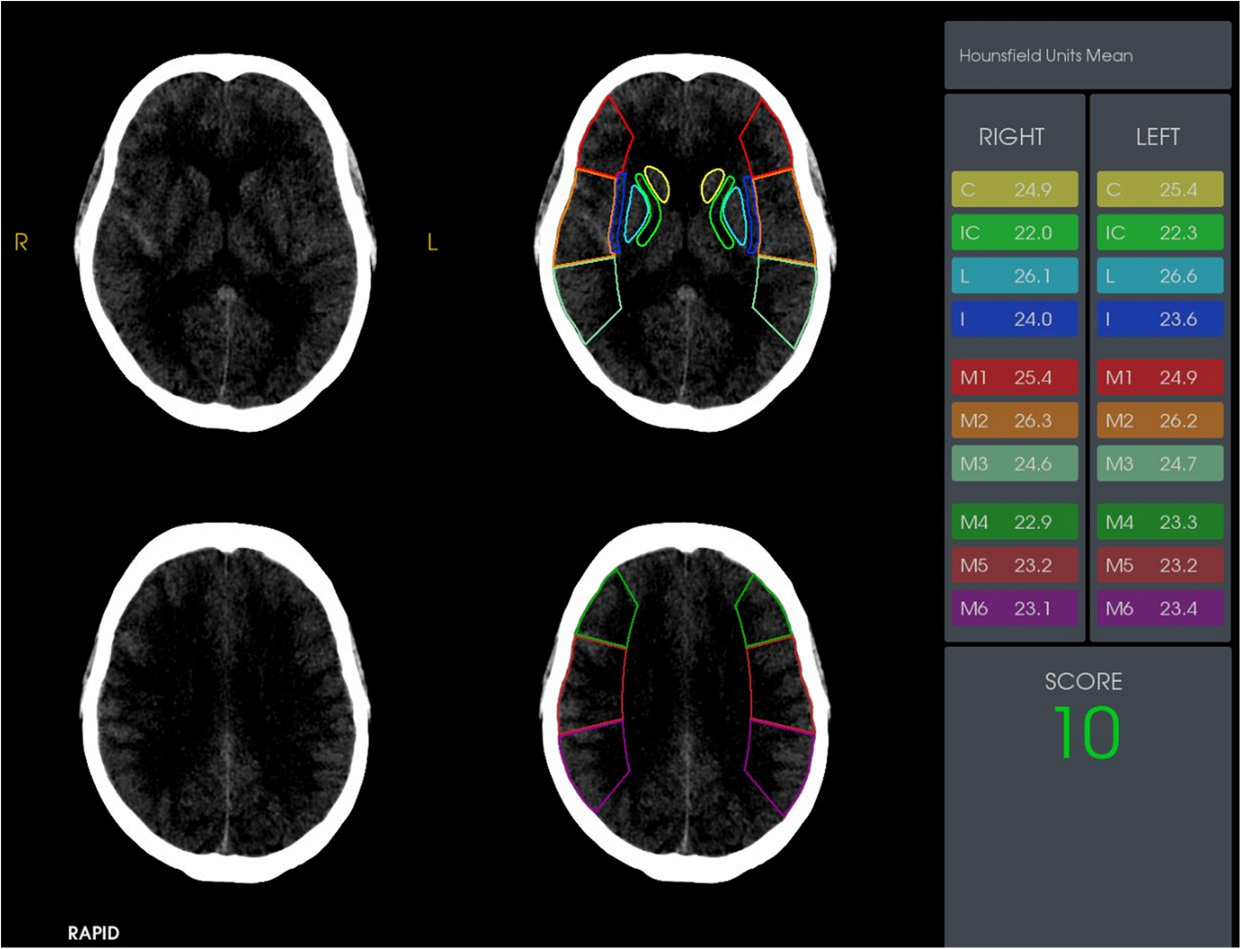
Imaging acquisition of RAPID‐ASPECTS.
